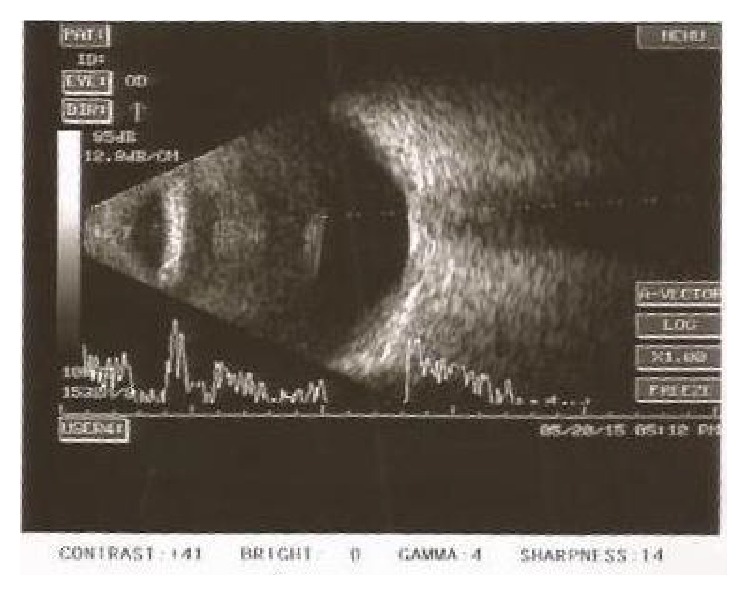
Right eye (Patient 2) B-scan ultrasosonography shows normal features suggesting resolution of choroidal detachment following withdrawal of chlorthalidone.
